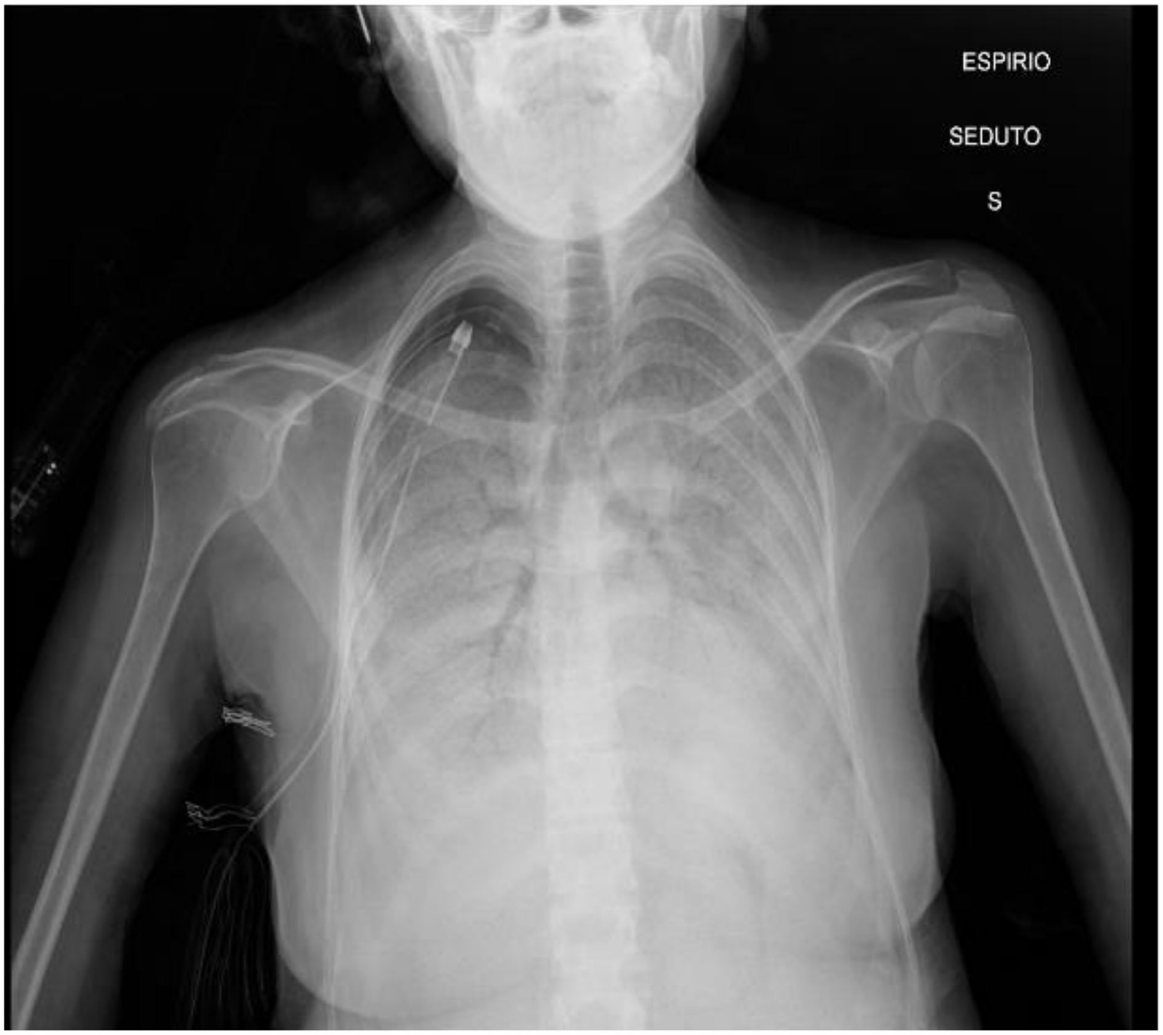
Chest X-ray on admission.
